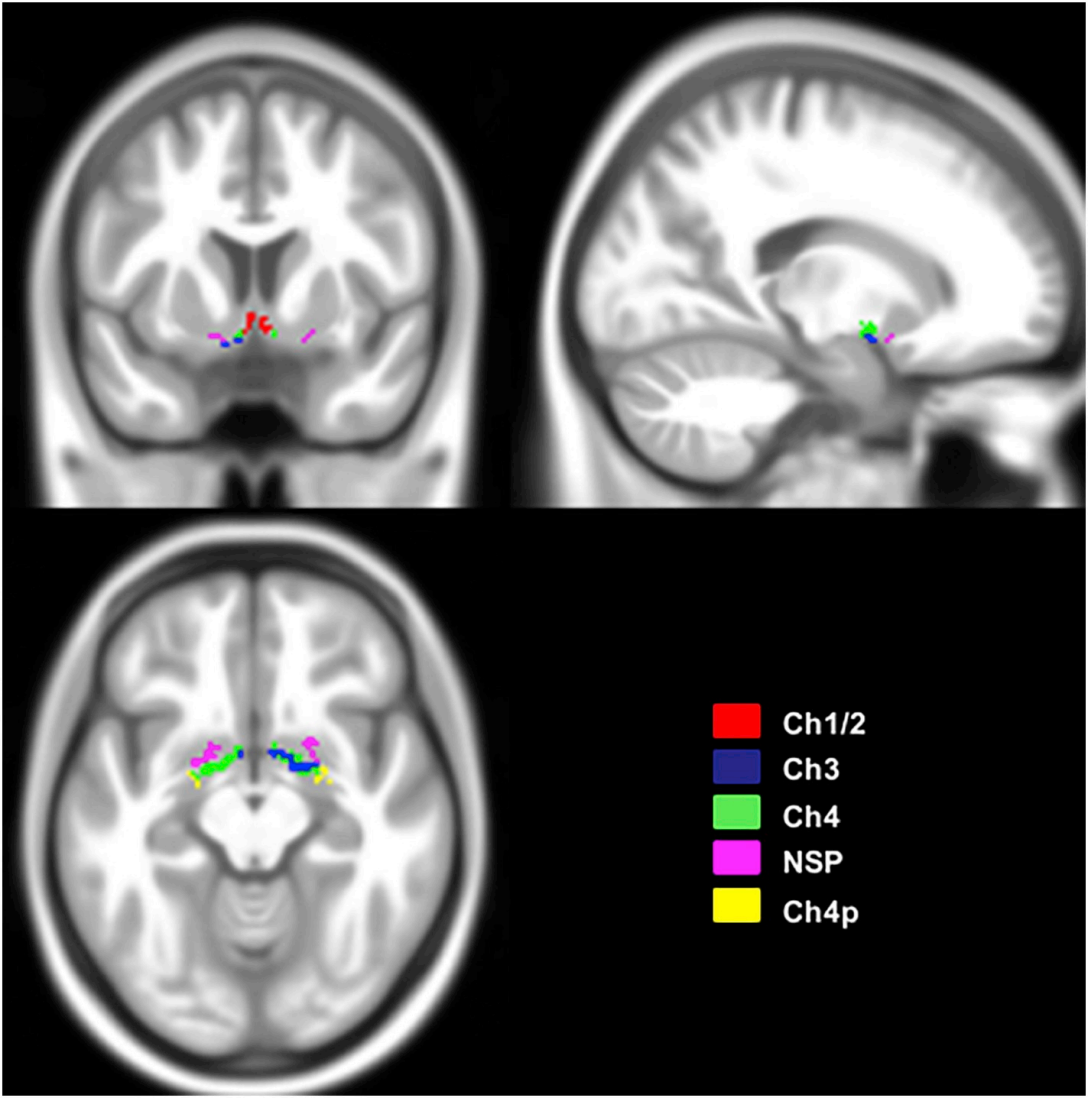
Outline of the anatomical position and extent of the cholinergic forebrain. Overlay of the cholinergic basal forebrain (chBFN) ROI's onto the T1-weighted MNI-template as described in detail by Kilimann et al., 2014. The different colors refer to the different nuclei. (For interpretation of the references to color in this figure legend, the reader is referred to the web version of this article.)
